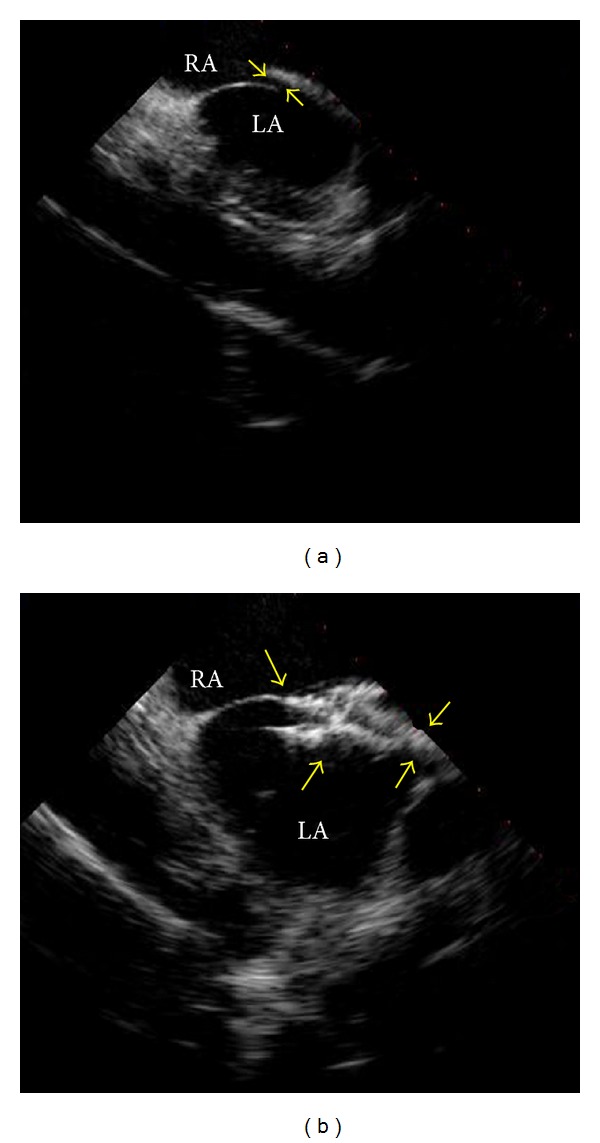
Intracardiac echocardiogram from patient 1 before and after PFO closure. (a) The intracardiac echocardiogram shows the right atrium (RA), left atrium (LA), and PFO (demarcated by arrows). (b) Intracardiac echocardiography following deployment of the closure device, also demarcated by arrows (28 mm CardioSEAL Septal Occluder; NMT Medical Inc., Boston, MA, USA).
